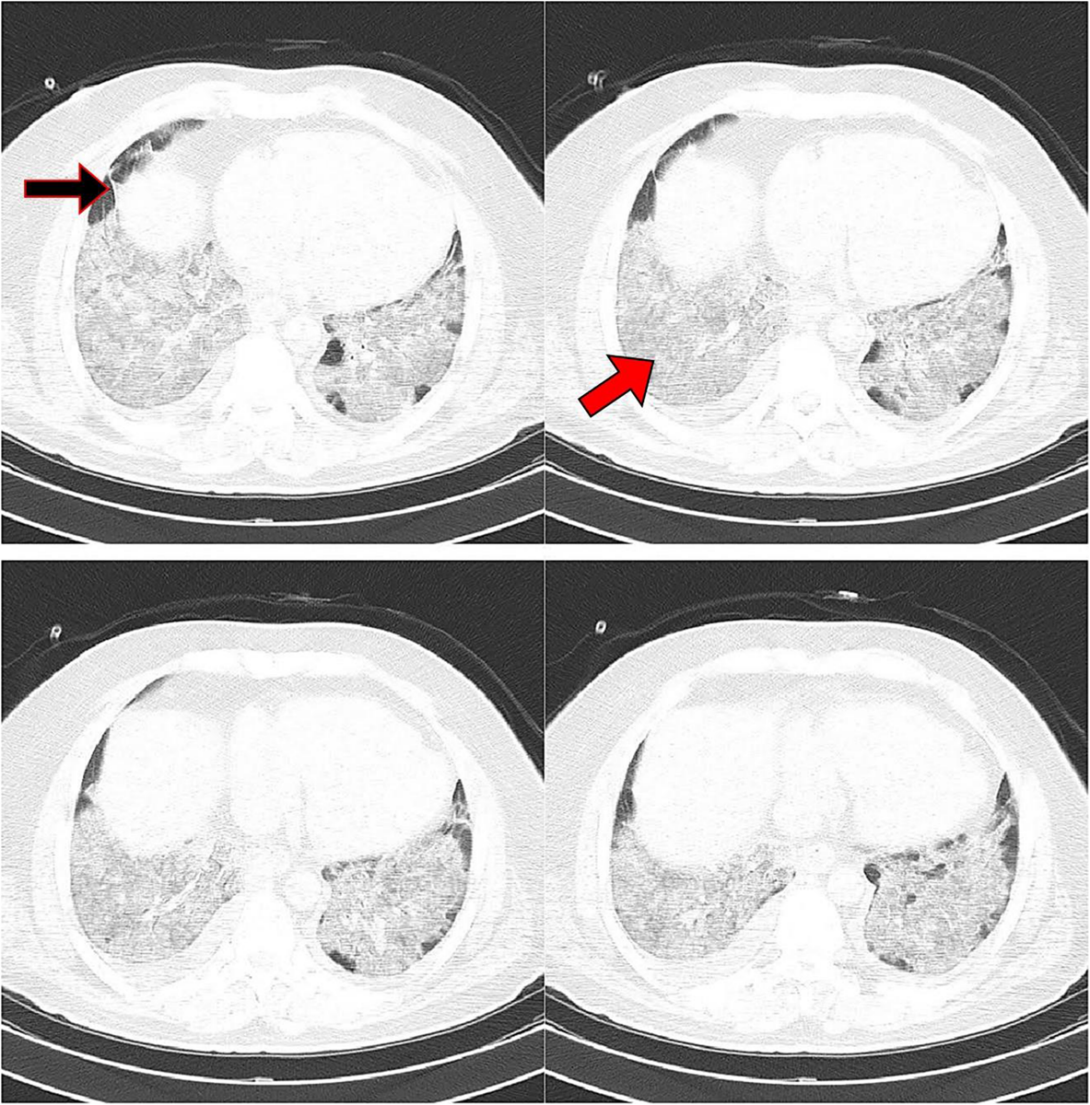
A 62-year-old male patient presented with fever, chills, myalgia, headache, and sputum discharge, who was admitted to ICU for 2 days and died. Extensive multilobar ground glass opacity and consolidation (red arrow), and parenchymal bands (black arrow) are observed in the CT scan, suggesting acute respiratory distress syndrome. The total CT severity score was calculated as 25, and the disease severity was critical
